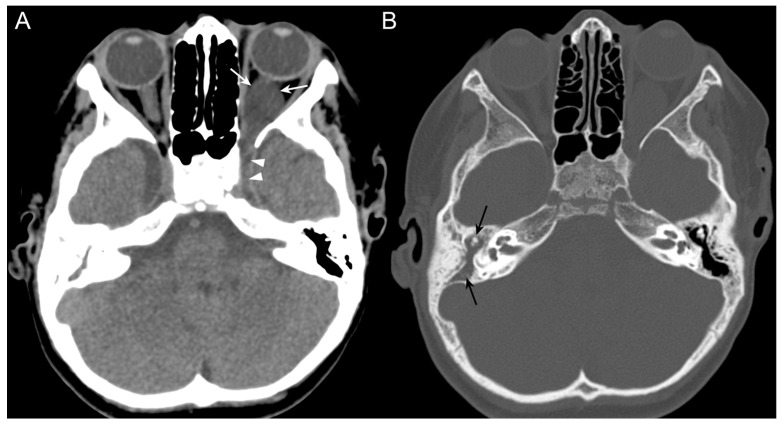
(A): CT without contrast agent administration shows an intra-orbital retrobulbar fluid-density sac (white arrows) on the left side. Posteriorly the fluid-filled sac replaces the fat tissue in the superior orbital fissure. The left cavernous sinus (arrowheads) is filled by a low density, similar to the intra-orbital sac. (B): the right middle ear is filled with fluid (black arrows).
